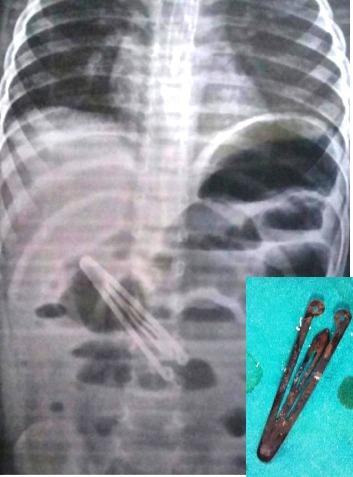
Figure 1: Hair clip seen in x-ray abdomen. Inset shows retrieved hair clip.
